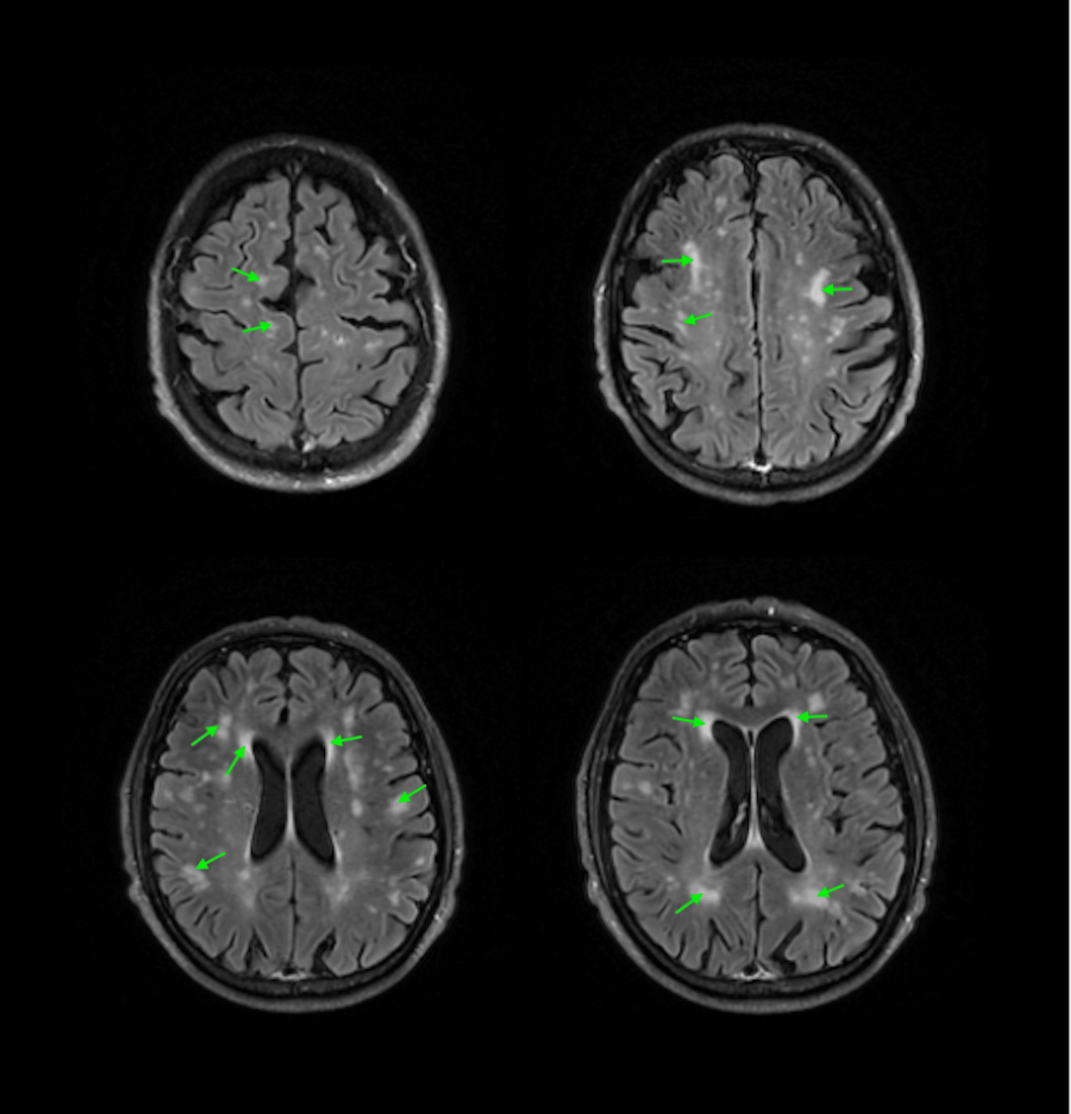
Fluid-attenuated inversion recovery (FLAIR) MRI showed multiple, partially confluent foci and hyperintense areas (marked with arrows) of various sizes located in the white matter of both cerebral hemispheres. Bilateral widening of the Virchow-Robin perivascular space was present. Notably, T1-weighted images showed normal signal intensity without focal lesions; diffusion-weighted imaging (DWI) sequence and corresponding apparent diffusion coefficient (ADC) maps revealed no evidence of restricted diffusion. The patient’s MRI findings were not consistent with the diagnosis of CJD. Typical MRI diagnostic features of this disease involve high intensity of the caudate nucleus, putamen, or at least two cortical regions (temporal, parietal, occipital), either on DWI or FLAIR sequences [13].
